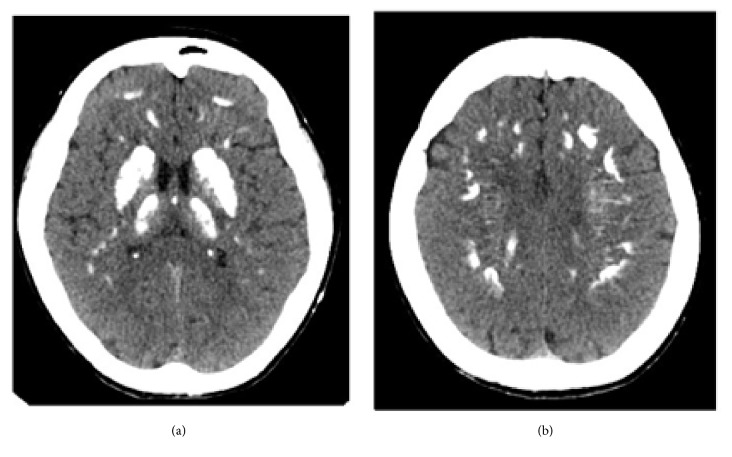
Basal ganglia (a) and subcortical (b) calcifications (CT scan).
